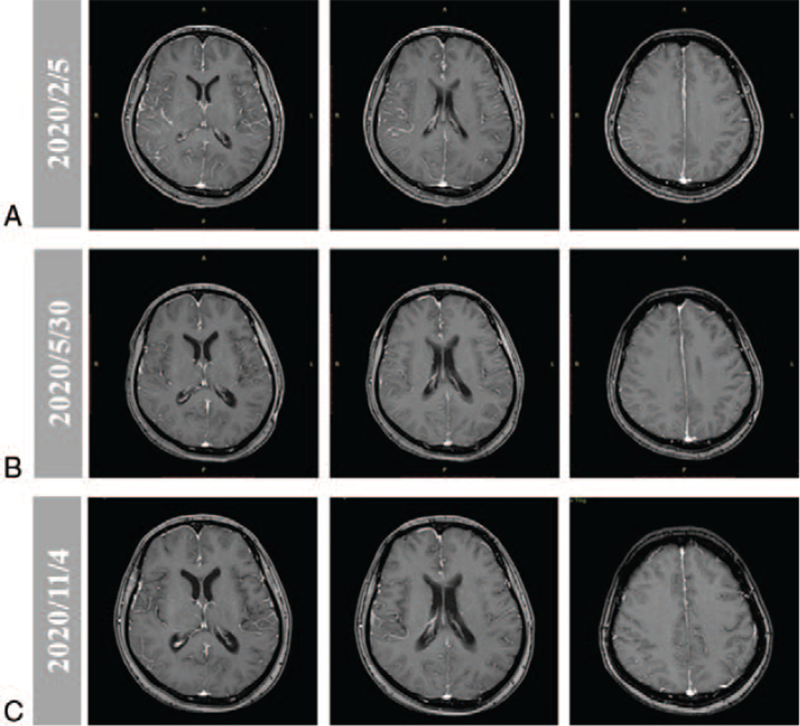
MRI image showed the changes of LM during treatment. (A) Disease progression after osimertinib a year and a half, line-like enhancement was seen in the brain. (B) Three months after erlotinib combined with bevacizumab and chemotherapy treatment, the line-like enhancement had significantly reduced. (C) Four months after erlotinib monotherapy treatment, the line-like enhancement was as before. LM = leptomeningeal metastasis, MRI = magnetic resonance images.
